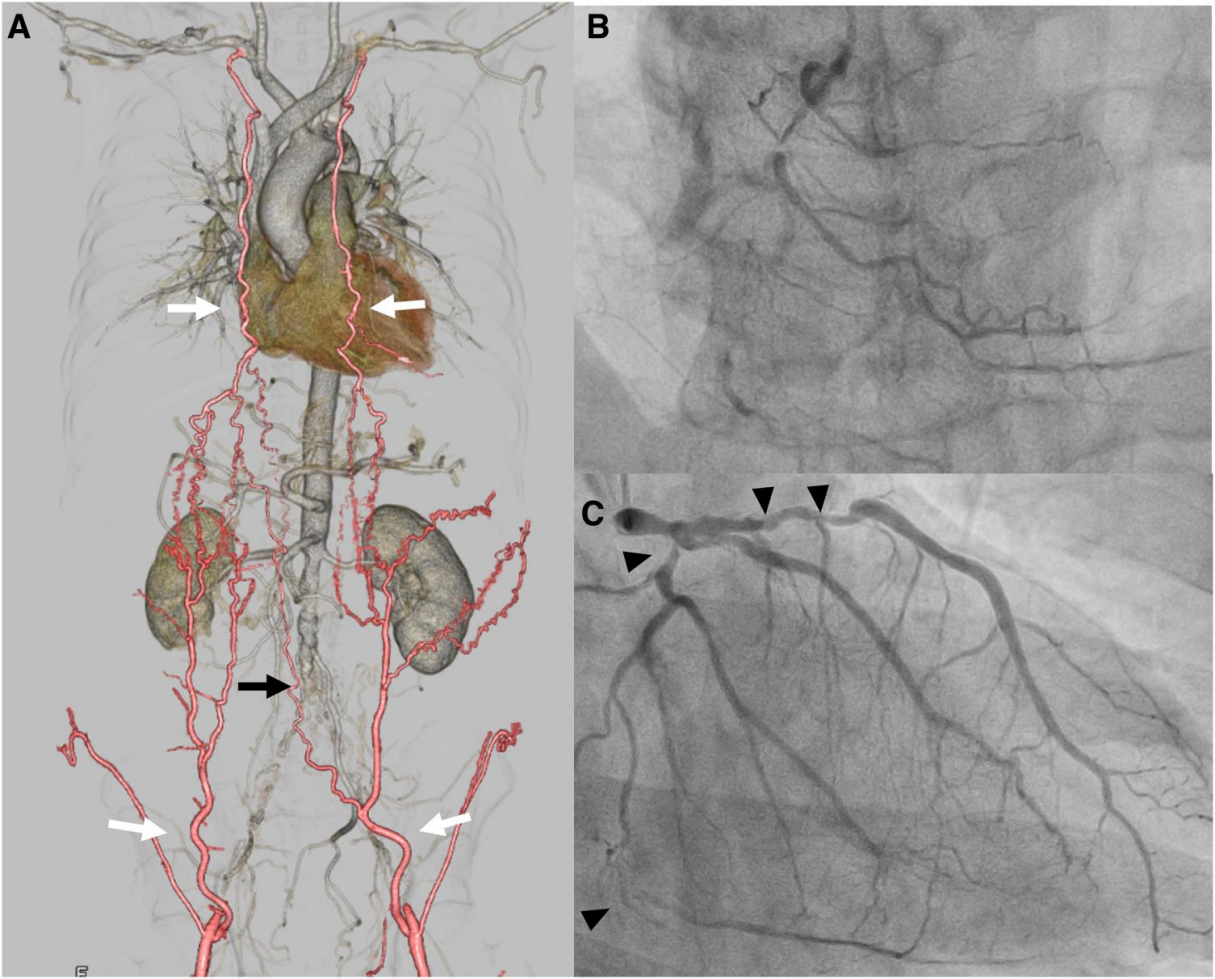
(A) 3D computed tomography showed the occlusion of infrarenal aorta and bilateral common iliac artery (black arrow). Inferior epigastric artery from ITA provided collateral flow to the lower extremities (white arrow). (B and C) Coronary angiography showed total occlusion of RCA and severe stenosis of LAD and LCX (arrow head). ITA: internal thoracic artery; LAD: left anterior descending artery; LCX: left circumflex artery; RCA: right coronary artery
